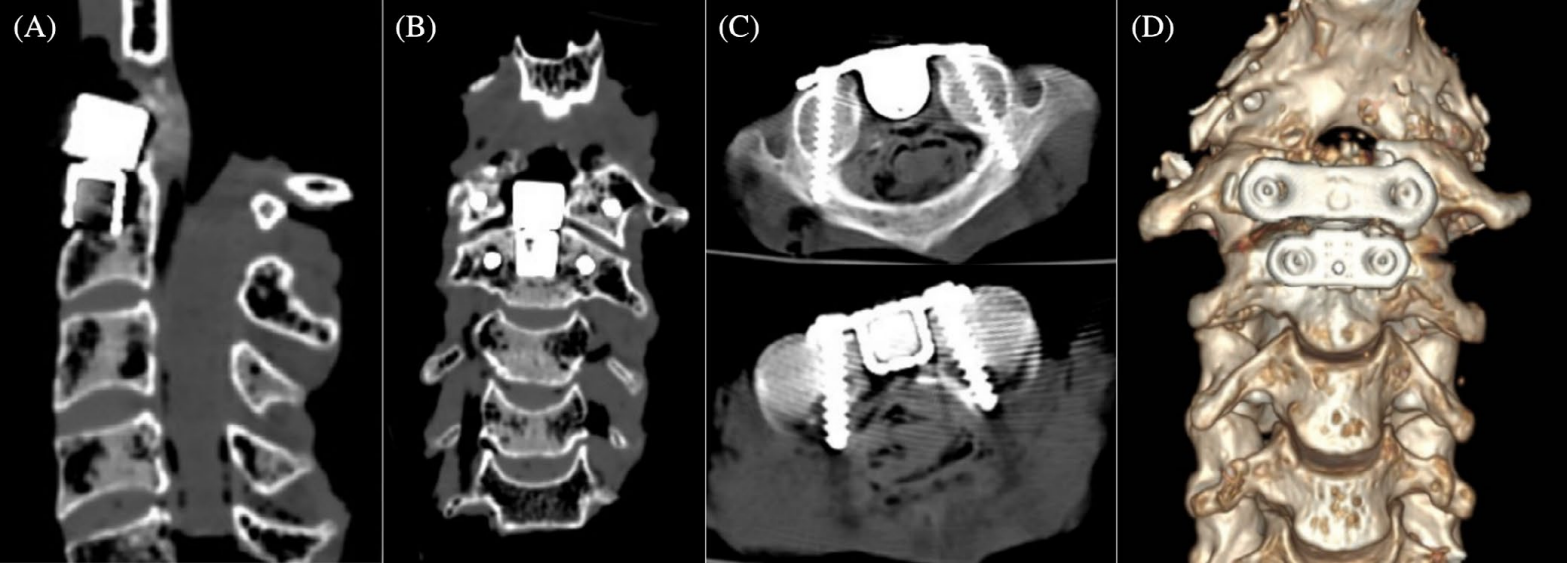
Postoperative CT scan and three‐dimensional reconstruction of the novel artificial atlanto‐odontoid joint (AAOJ) replacement (A) Sagittal view; (B) Coronal view; (C) Axial view of the atlas and axis; (D) Three‐dimensional reconstructed image.
